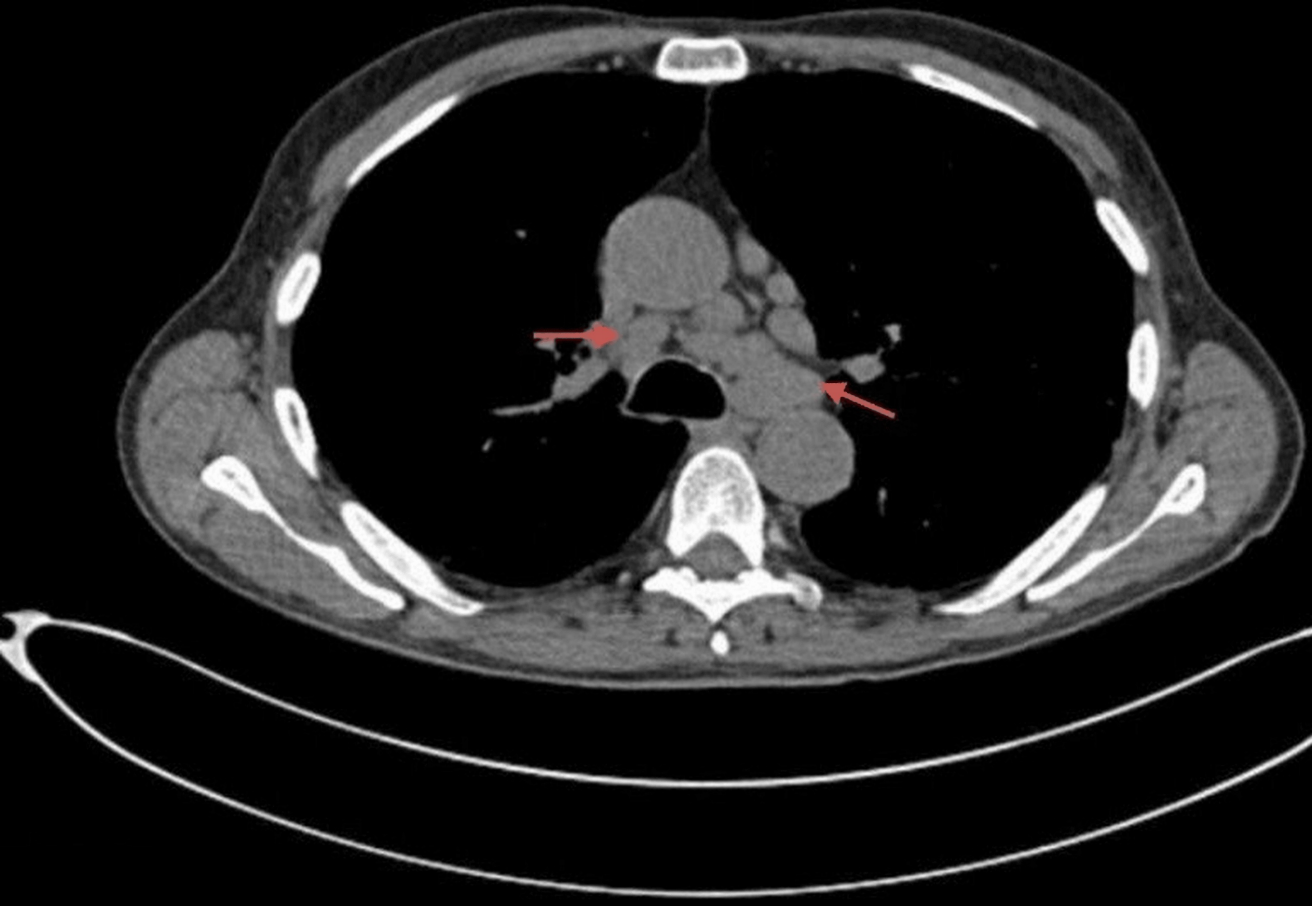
Non-contrast CT thorax. Technique: Non-contrast computed tomography (CT). Findings: Persistent fibrotic interstitial lung changes. Within the limitations of a non-enhanced study, no stigmata of malignancy were observed. Conclusion: Indeterminate mediastinal hilar lymph nodes.
